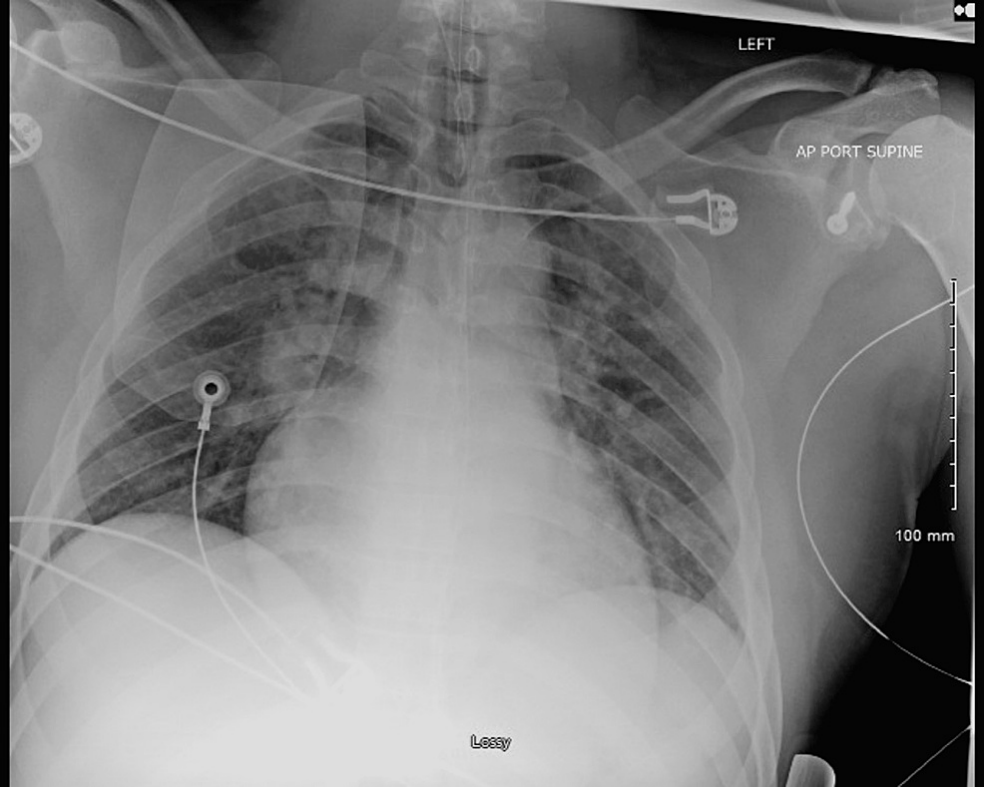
Chest X-ray post-intubation in the emergency department, notable for mild central pulmonary vascular congestion.
